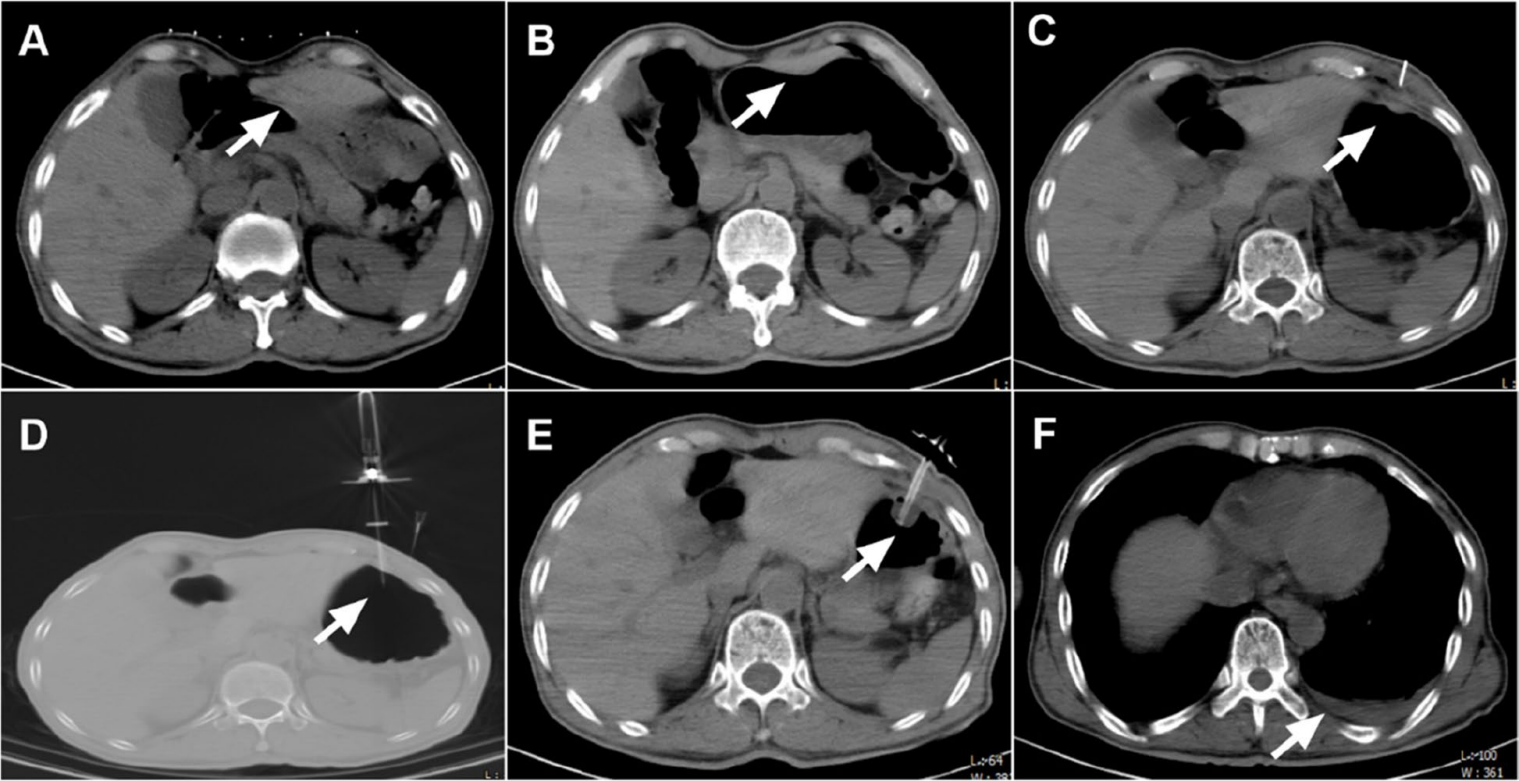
A 59-year-old male patient with esophageal squamous cell carcinoma and esophageal obstruction. Preoperative CT showed complete obstruction of the gastric cavity by the left hepatic lobe (A). Despite insufflation of the gastric cavity, the proposed site for rectus abdominis stoma placement was still obstructed by the left hepatic lobe (B). Therefore, the decision was made to place the stoma at the left 6th intercostal space (C). A 20-G gastric wall fixator was used to secure the gastric wall (D). Following wall fixation, a 16-Fr support sheath was inserted to facilitate fistula creation, and a 15-Fr gastric catheter was inserted through the opening (E). Postoperative CT revealed minimal bleeding in the left thoracic cavity (F)
